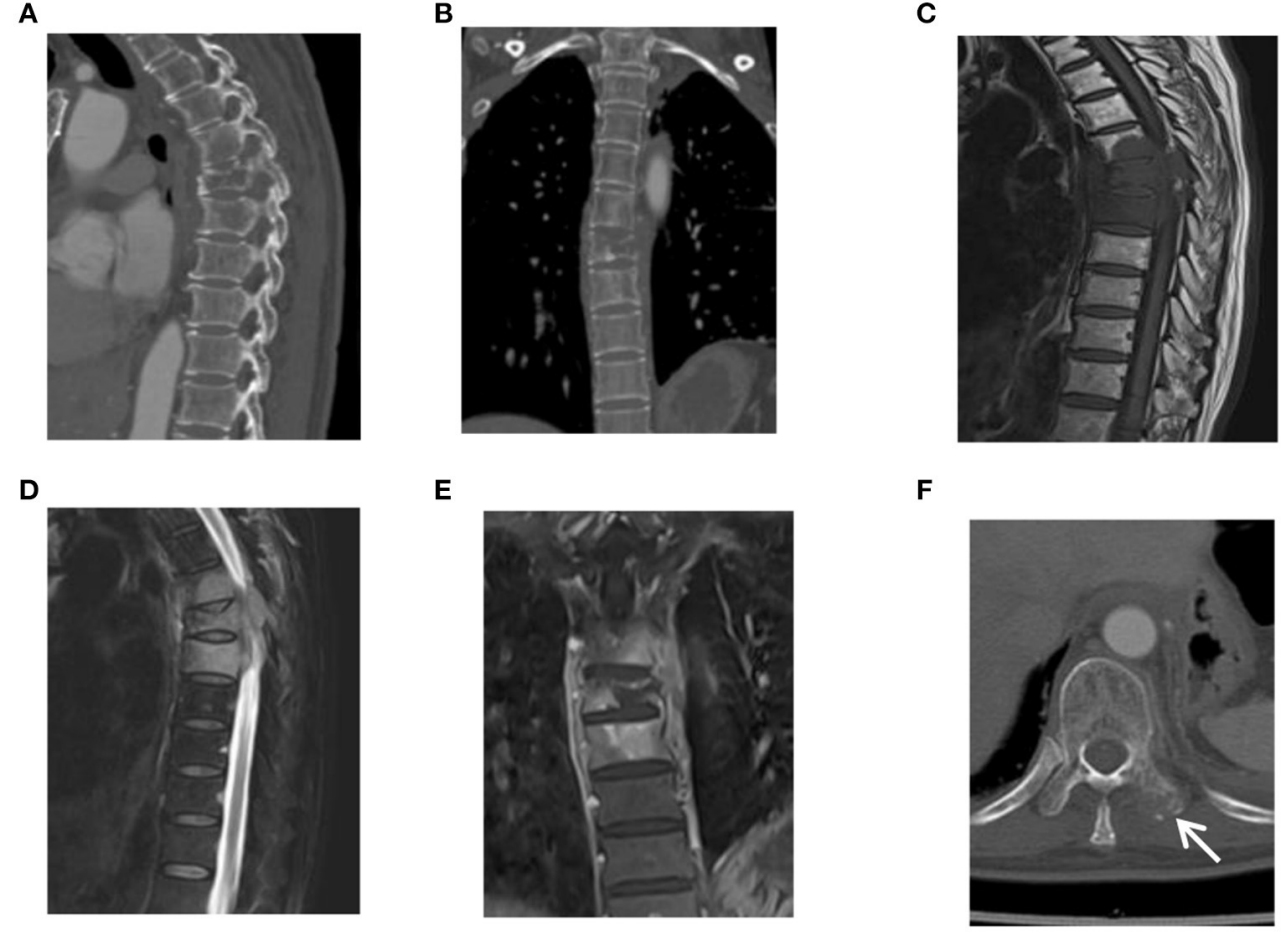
CT and MRI of a 49-year-old female patient with mediastinal EHE before the second treatment. (A–D) CT (A,B), and MRI (C,D) revealed multifocal lesions of the thoracic vertebrae 5–7. (E) An enhanced MRI of the venous phase revealed multifocal lesions of the thoracic vertebrae with obvious asymmetrical enhancement. (F) The CT indicated a local ruin of the left transverse process of the 10th thoracic vertebra (arrow).
